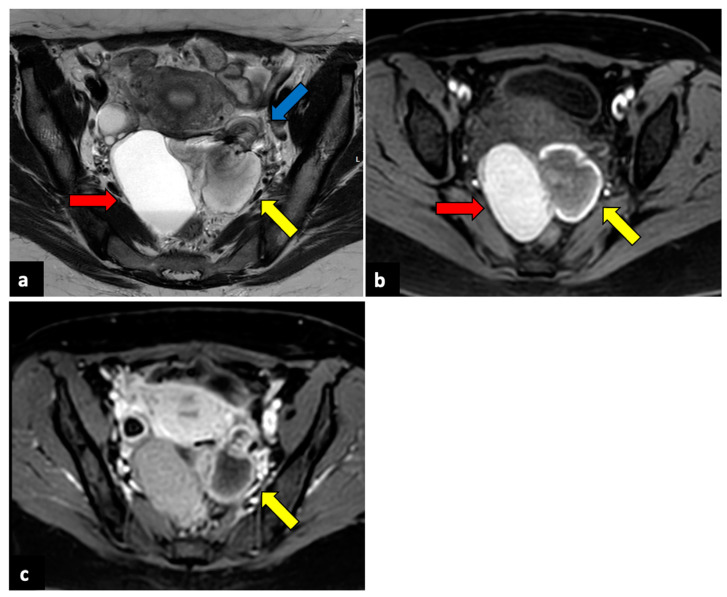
Left ovarian torsion in a thirty-nine-year-old patient with acute pelvic pain. Axial T2-weighted (a) and T1-weighted fat saturated before (b) and after (c) gadolinium MR pelvic images show an enlarged left ovary located in the Douglas pouch (yellow arrows), with and adjacent twisted vascular pedicle (“whirlpool sign”, blue arrow). The ovary presents a peripheral high signal on T1 extending to the pedicle (rim of methaemoglobin) without enhancement upon post-contrast sequences. A large cystic lesion arises from the left ovary with an internal high signal on both T1 and T2 (red arrows) and declivous sediment of low signal on T2, suggestive of a haemorrhagic cyst. The patient underwent a laparoscopic left adnexectomy, and pathology confirmed the diagnosis of ovarian torsion possibly due to the haemorrhagic cyst.
